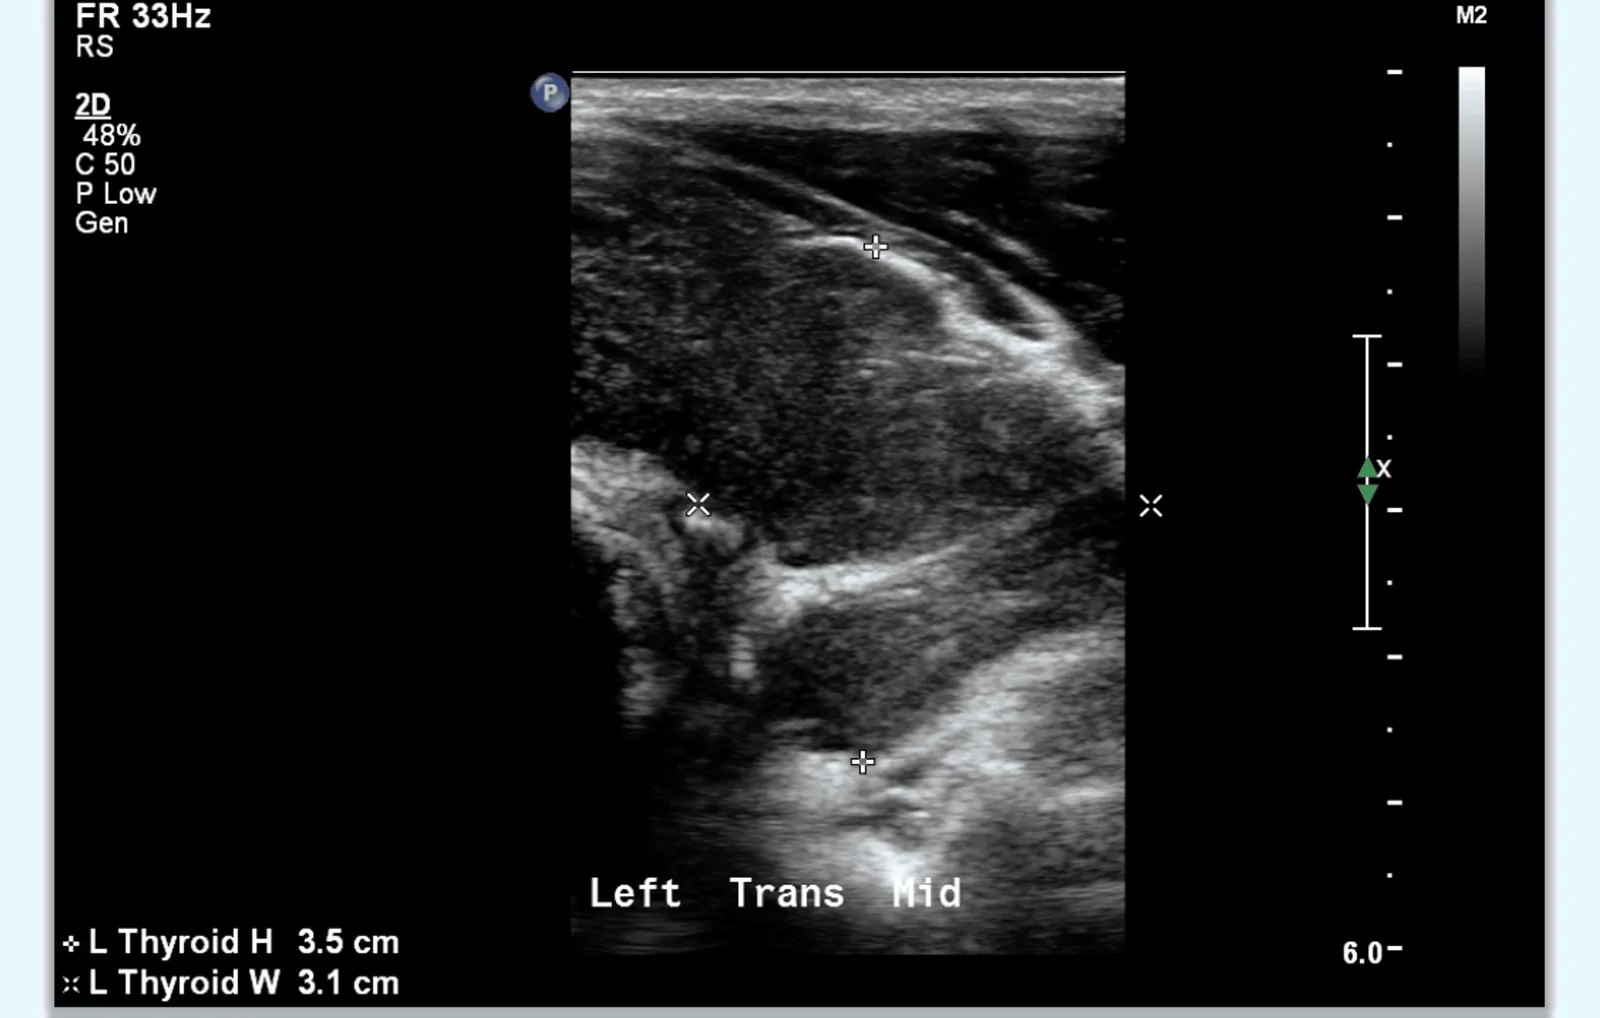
Transverse ultrasound view of the left lobe of the thyroid, measuring 3.5 x 3.1 cm.
